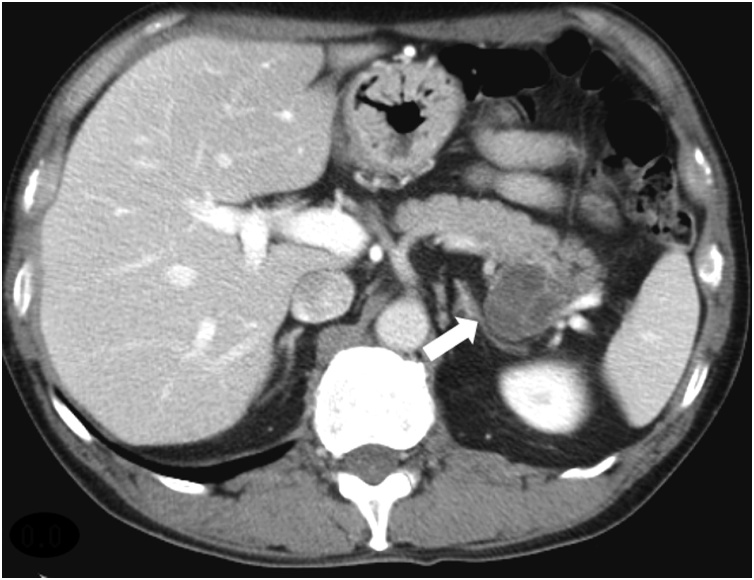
Computed tomography (CT): Enhanced CT showed enhancement of the cystic wall and septa while the cystic contents appeared unenhanced (arrow).
